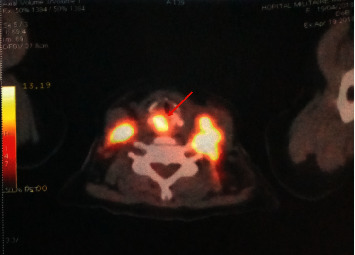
PET/CT at diagnosis showed pathological laryngeal hypermetabolism with cricoid cartilage involvement (red arrow).
